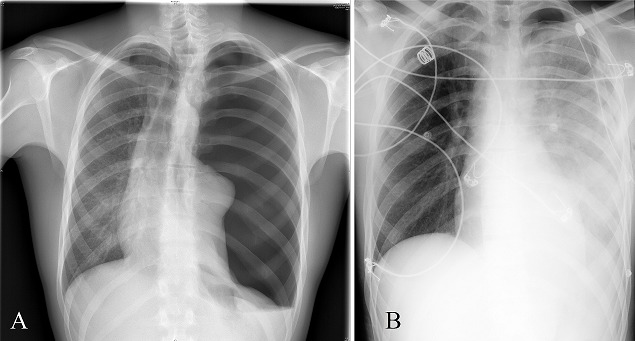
(A) left tension pneumothorax with midline structures desviation to the right; (B) postprocedure chest Xray showed near-immediate reexpansion of the left lung with new infiltrates in the whole lung
